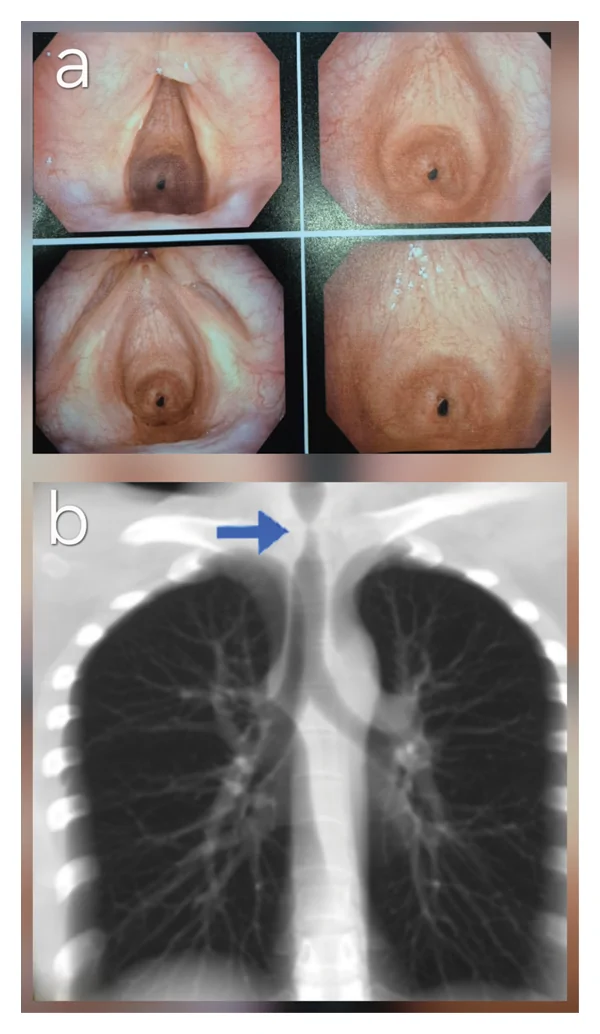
(a) Fiberoptic laryngoscopy and (b) high‐resolution CT of neck and thorax with three‐dimensional reconstruction of the airway showing tracheal stenosis in cervical trachea.
